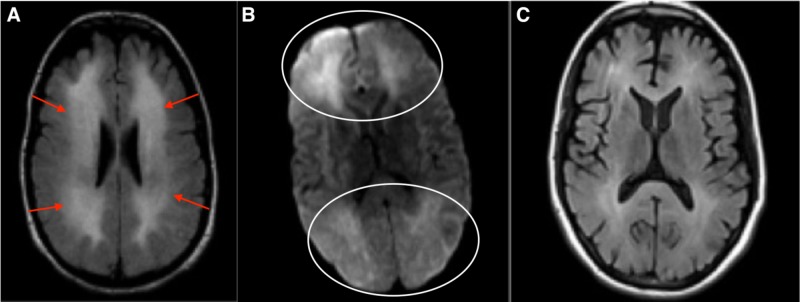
A. FLAIR, B. Diffusion, C. Follow-up MRI after one year. (A) shows increased FLAIR signal in the cerebral white matter (arrows). (B) shows diffusion restriction both anteriorly and posteriorly (oval circles). (C) shows improvement in the T2/FLAIR hyperintensities one year after the initial brain imaging. FLAIR: Fluid-attenuated inversion recovery; MRI: Magnetic resonance imaging.
